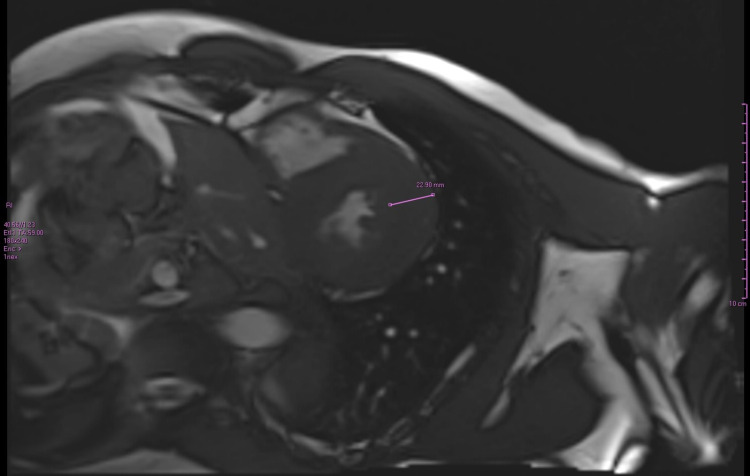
Cardiac MRI of the patient Cine image of the short oblique-axis of the left ventricle. The thickness of the basal anterior lateral wall (purple arrow span) is measured at 23mm (normal values of 6.5mm - 8.5mm), demonstrating significant myocardial thickening.
